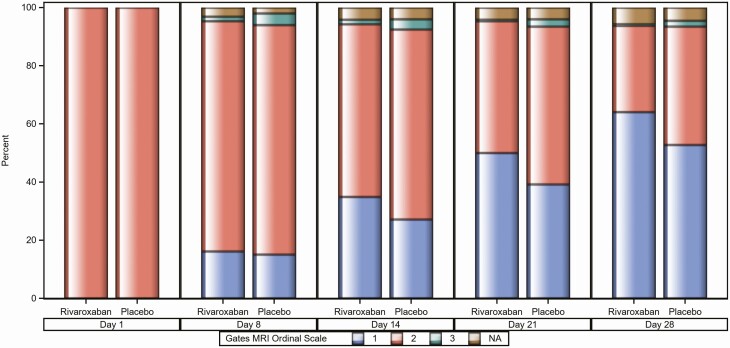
Proportion of participants within each Gates Medical Research Institute scale at days 1, 8, 14, 21, and 28 (modified intention-to-treat [mITT] population). Gates MRI ordinal scale: 1 = asymptomatic/symptoms similar to pre-coronavirus disease 2019 (COVID-19) status, 2 = mild, 3 = moderate or severe, 4 = critically ill, 5 = critically ill with invasive mechanical ventilation or extrapulmonary complication, 6 = critically ill with extracorporeal membrane oxygenation, and 7 = death. NA = Participants in mITT population who had no available Gates MRI scale value at post-baseline assessments.
